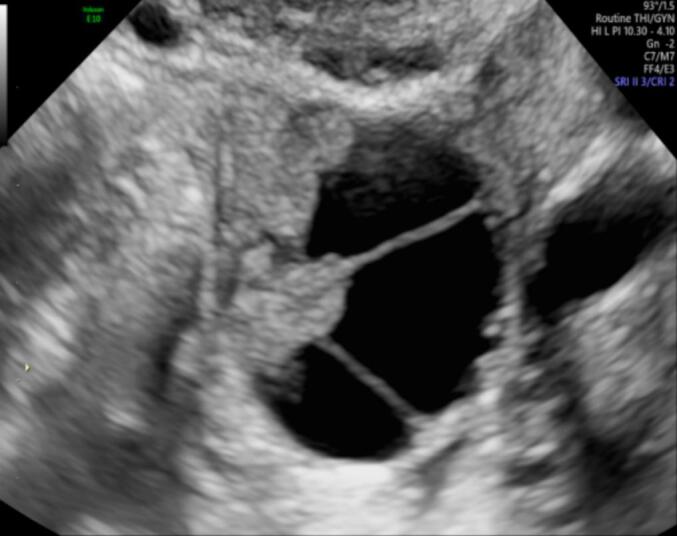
Ultrasonographic appearance of the mass.
